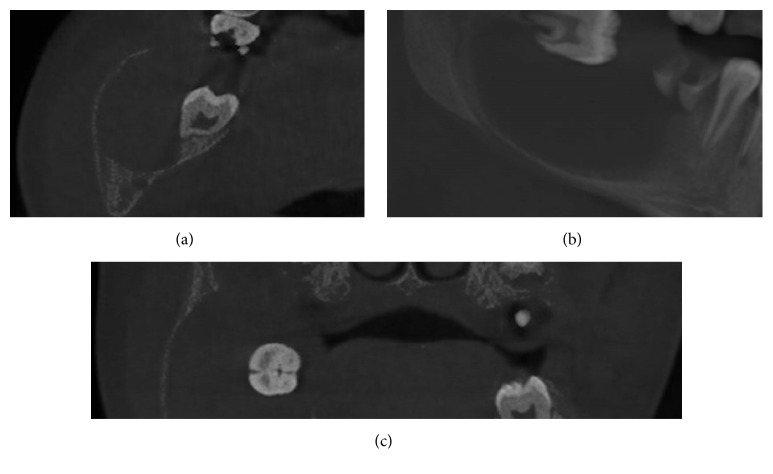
CBCT images showing axial (a), sagittal (b), and coronal (c) slices demonstrating the expansive aspect of the lesion and its internal homogeneous appearance.
